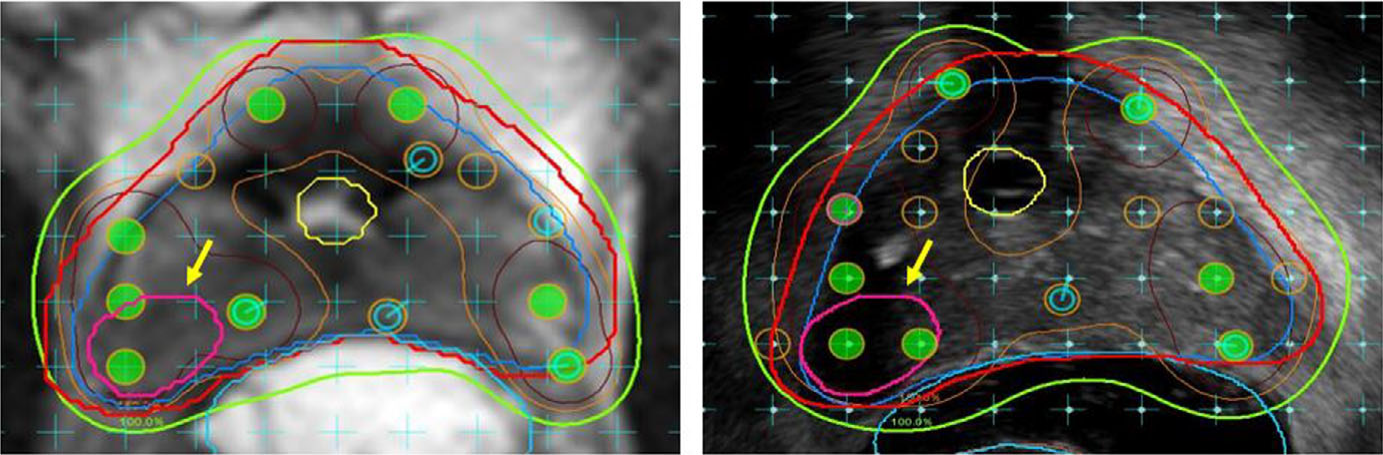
Targeted dose escalation of mpMRI‐identified PIRADS 5 lesion. Left: Preplan on T2 MRI sequence. Right: Intraoperative MRI/iTRUS fusion for real‐time planning. Contours: Royal blue, prostate; Red, PTV expansion; Yellow, Urethra; Magenta/Yellow arrow, PIRADS 5 lesion; Light blue, hydrogel spacer between prostate and rectum. Isodose lines: Green, 100% of prescription; Orange, 150%; Maroon, 200%.
